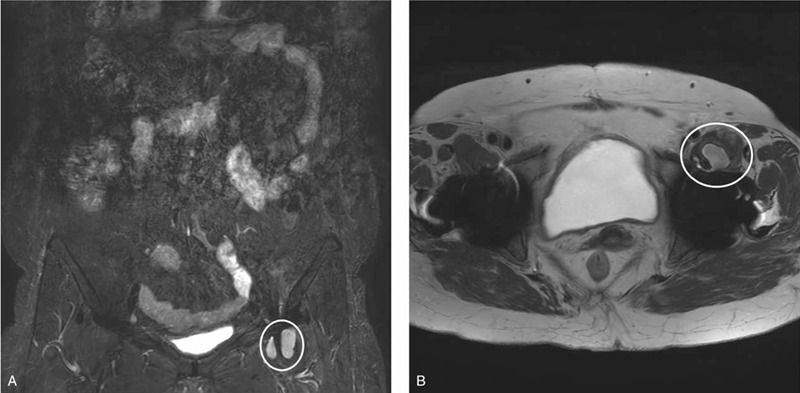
Enhanced lumbosacral plexus magnetic resonance imaging (MRI) scan of the left iliopsoas bursitis or hematoma (1.9 cm in thickness). The displacement of left femoral nerve due to iliopsoas bursitis/hematoma (white circle). (A) Coronal view of the T2-weighted MRI scans of the lumbosacral plexus. (B) Sagittal view of T2-weighted MRI scans of the lumbosacral plexus.
